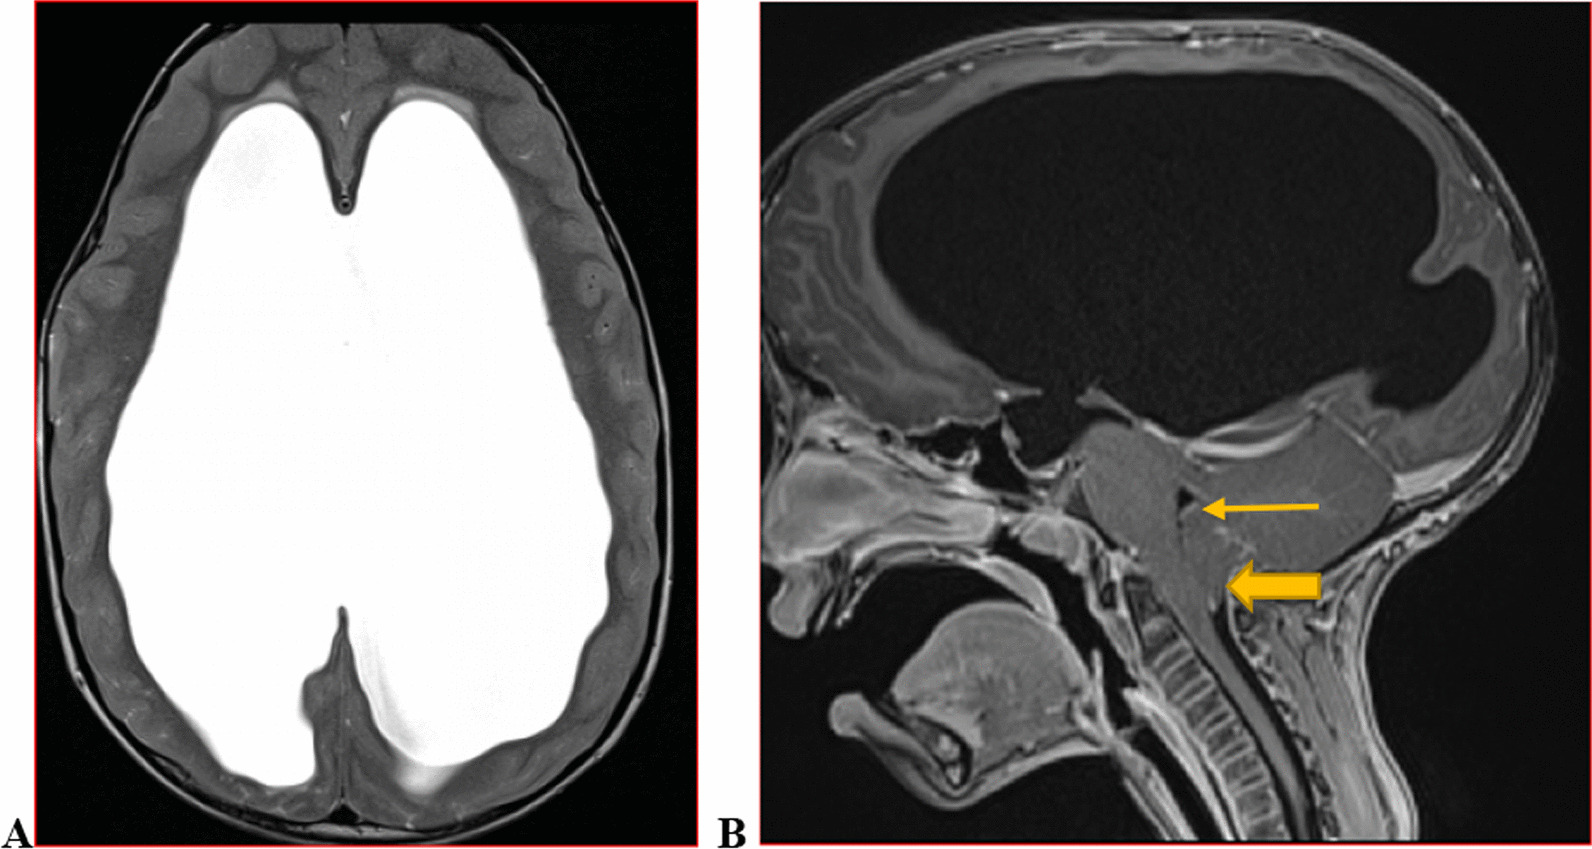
Nine year old male with increased head circumference and epilepsy since early childhood T1 and T2-weighted axial brain MRIs demonstrate grossly dilated lateral ventricles with absent interventricular septum (A); 4th ventricle is spared (arrow). Sagittal T1-weighted image (B) shows compression of the cerebellum9narrow arrow) with tonsillar herniation(wide arrow). This is a case of hydrocephalus due to aqueductal stenosis with elevated ICP
